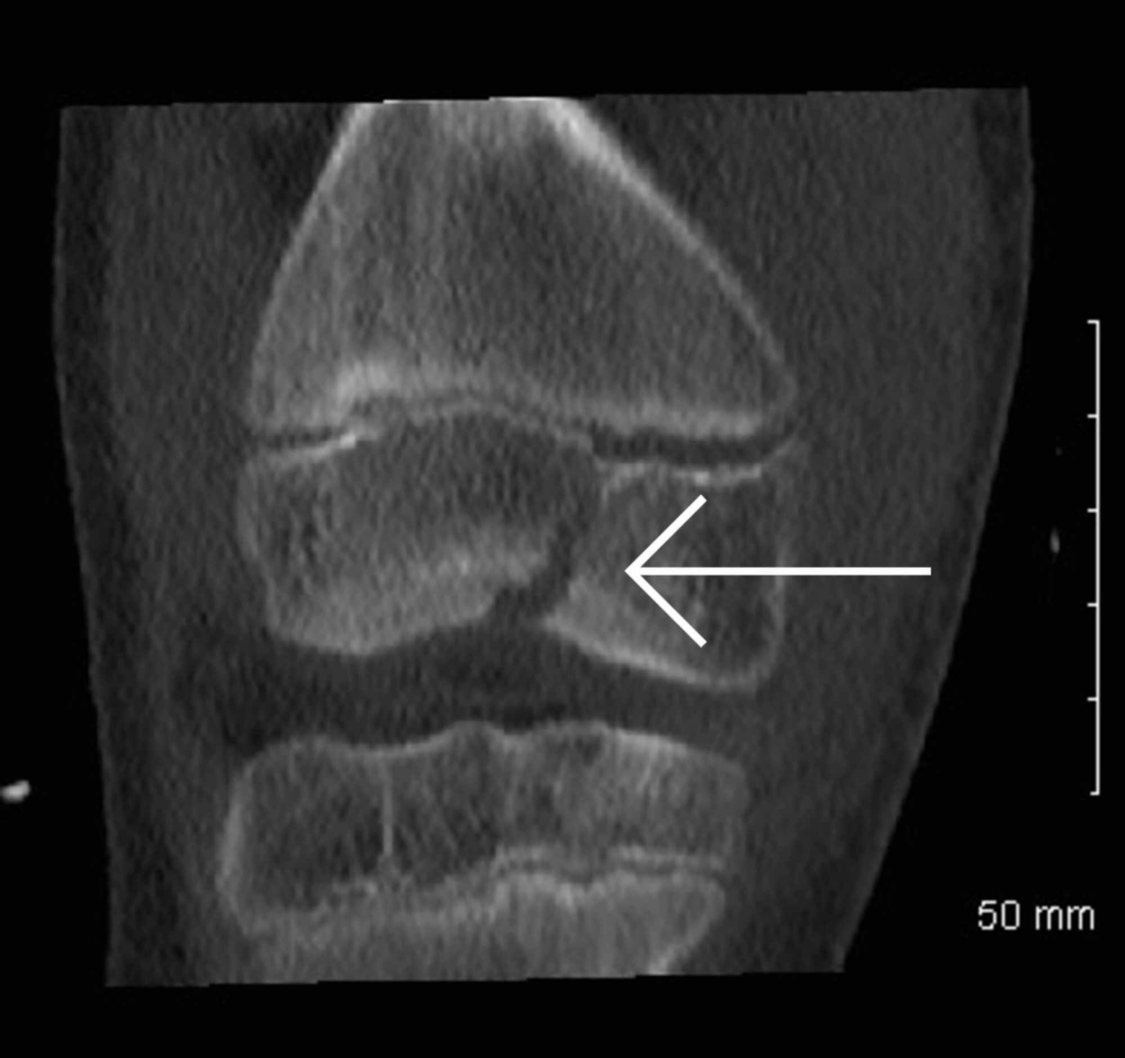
Coronal view of computed tomography scan of right knee post-injury
